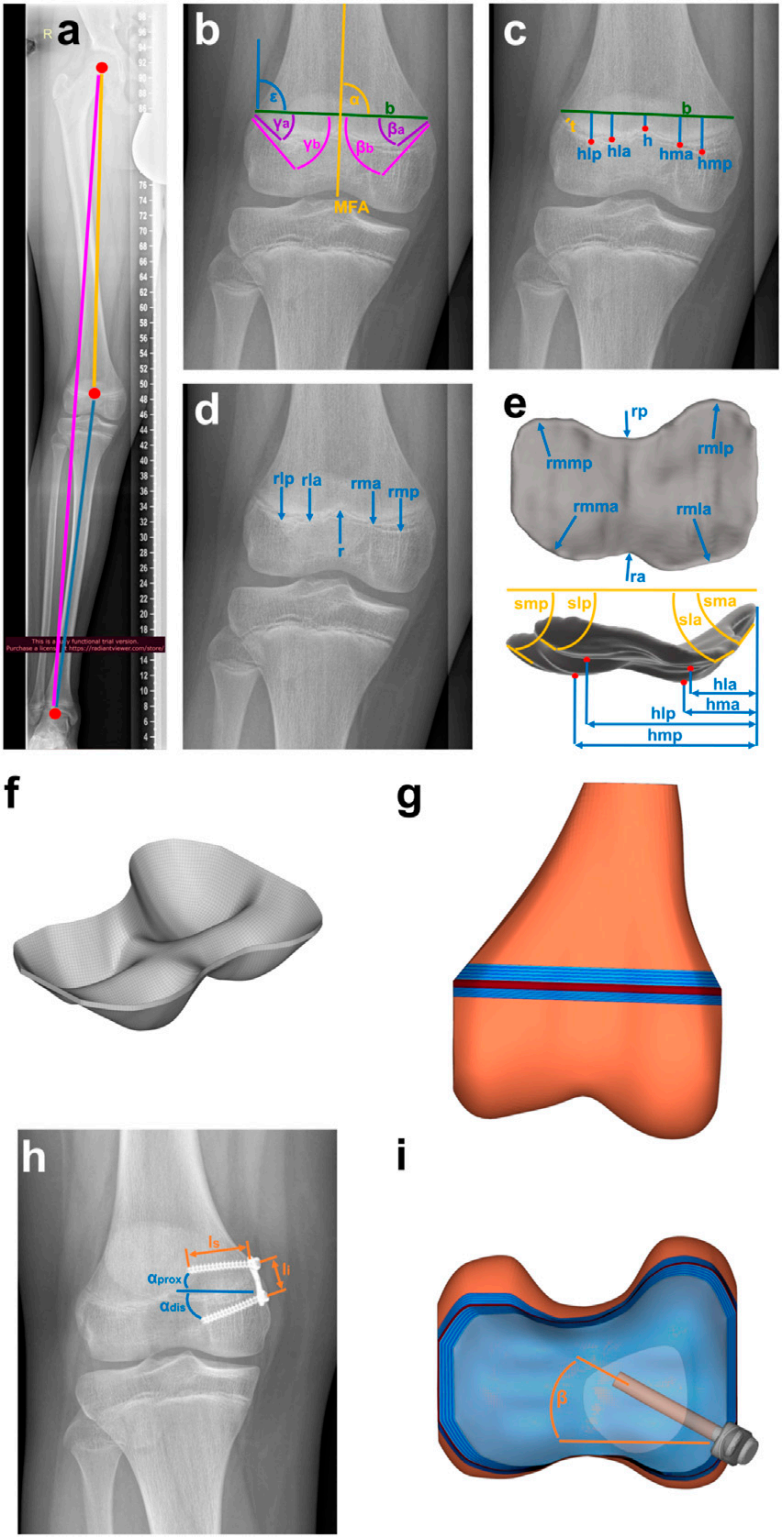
Creating the geometry based on clinical data: (A–E) Characteristic parameters of the growth plate and the knee, such as (A) malalignment, (B) edge steepness measured with angles medially β′ and laterally,γ′ each anterior,a’ and posterior, p’, (C) location of vertices, h’ laterally, l’ and medially, m’ each anterior, a’ and posterior, p’, width, b’ and thickness, t’, (D) radii, r’ laterally, l’ and medially, m’ each anterior, a’ and posterior, p’ and (E) additional parameters from 3D-MRI-data (Lustig und Vasanawala, 2022) such as the radii in the transversal plane, rm’, r’ laterally, l’ and medially, m’ each anterior, a’ and posterior, p’. This leads to (F) the 3D-Mesh of growth plate, implemented in (G) FE-Mesh of distal femur. (H) Implant parameters such as screw length, l_S’_, implant length, li’ and screw angles,α_prox_’, α_dis_’ are measured and implemented in (G) FE-Mesh of the distal femur with (I) the implant included.
